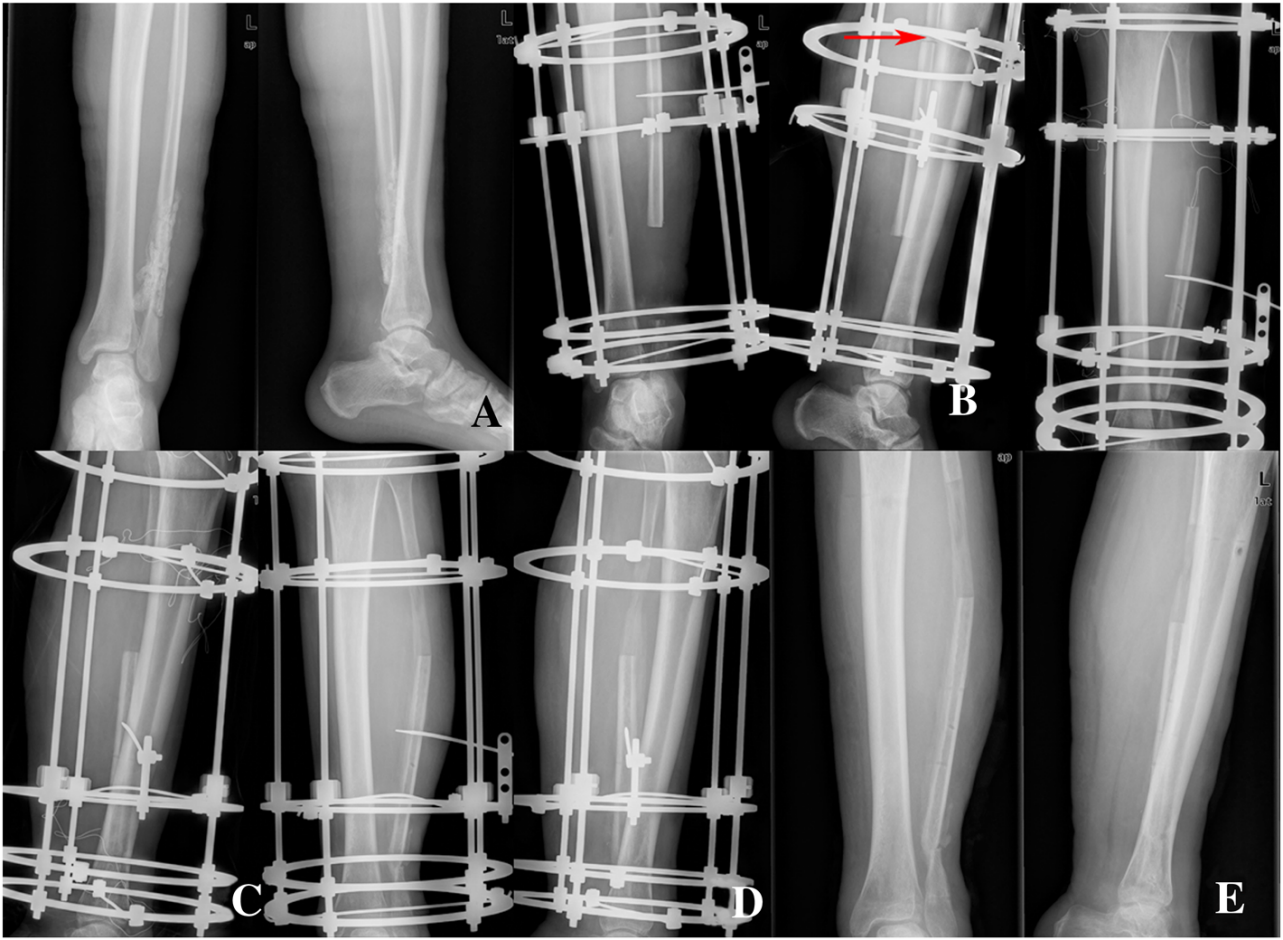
A 20-year-old man with osteomyelitis of the left fibular was treated by Ilizarov bone transport. a Radiograph of a 20-year-old man (case 1) who had osteomyelitis of the left fibular. b Debridement of the site of infected nonunion with 10cm bone defect in fibular and corticotomy of fibular (red arrow) after application of the fixator. c Four months after operation with bone transport, bone ends contacted at the docking site. d Nine months after operation, it showed good consolidation of the regenerate, and bone union at the docking site was obtained by bone grafting. e The external fixator was removed after nine months of the surgery, and it showed complete bone union
